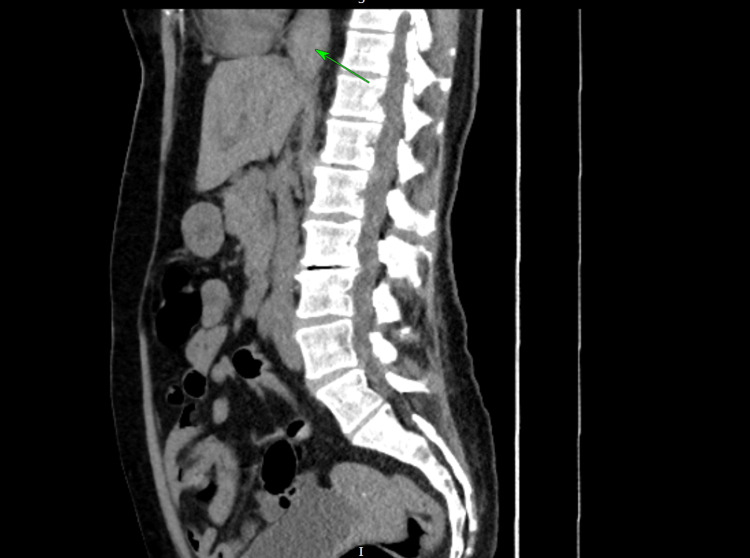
Computed tomography (CT) showing an oesophageal haematoma as pointed at by the green arrow (sagittal view)
